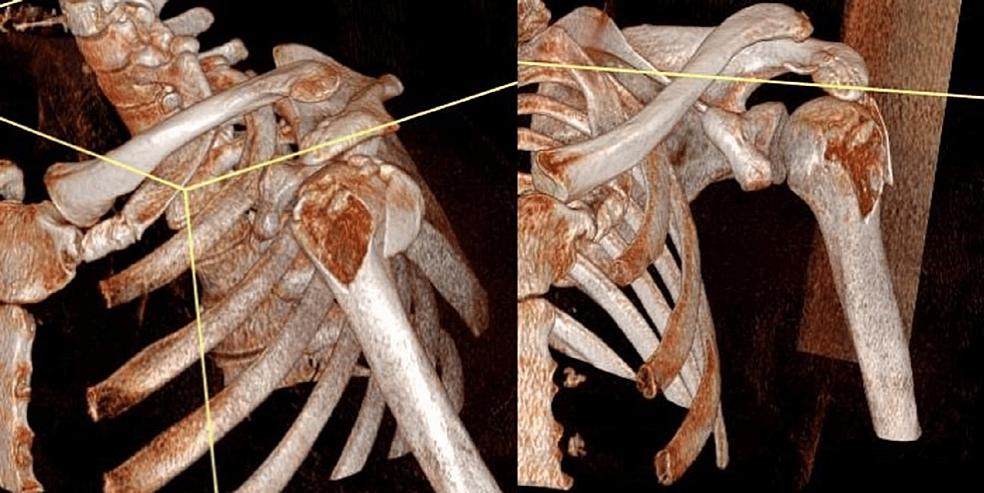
Three-dimensional reconstruction images from the patient's CT scan These give a better impression of the significant displacement of the greater tuberosity fragment and serve to demonstrate how deceptive plain radiographs can be, if not interpreted carefully and with a high index of suspicion. CT: Computerized tomography
